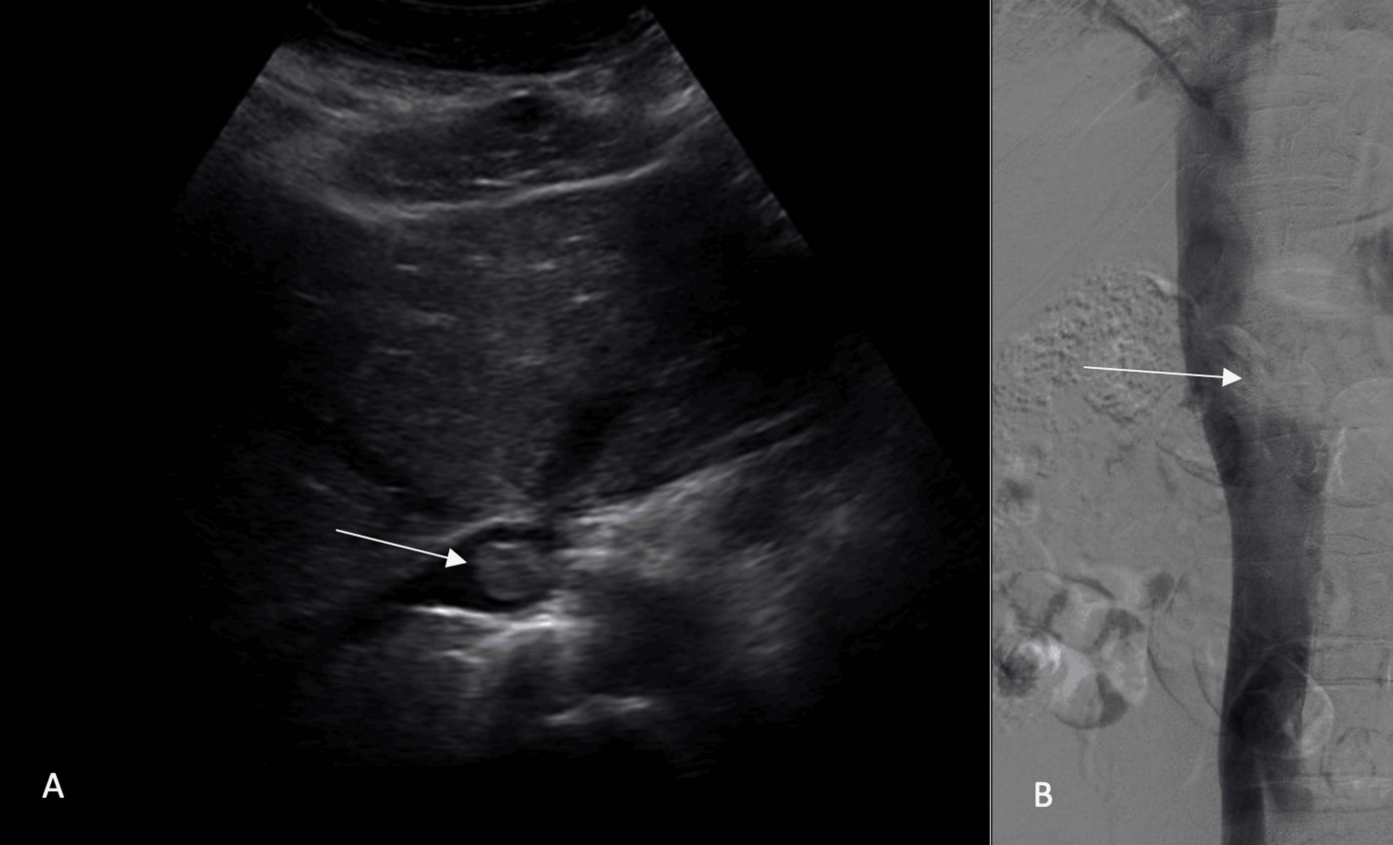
Imaging findings supporting the diagnosis of a lesion in the IVC A) Abdominal ultrasound demonstrating a nonocclusive echogenic structure (marked by the arrow) in the lumen of the upper abdominal IVC, B) Venocavogram showing a filling defect in the upper abdominal IVC (marked by the arrow) IVC: inferior vena cava
